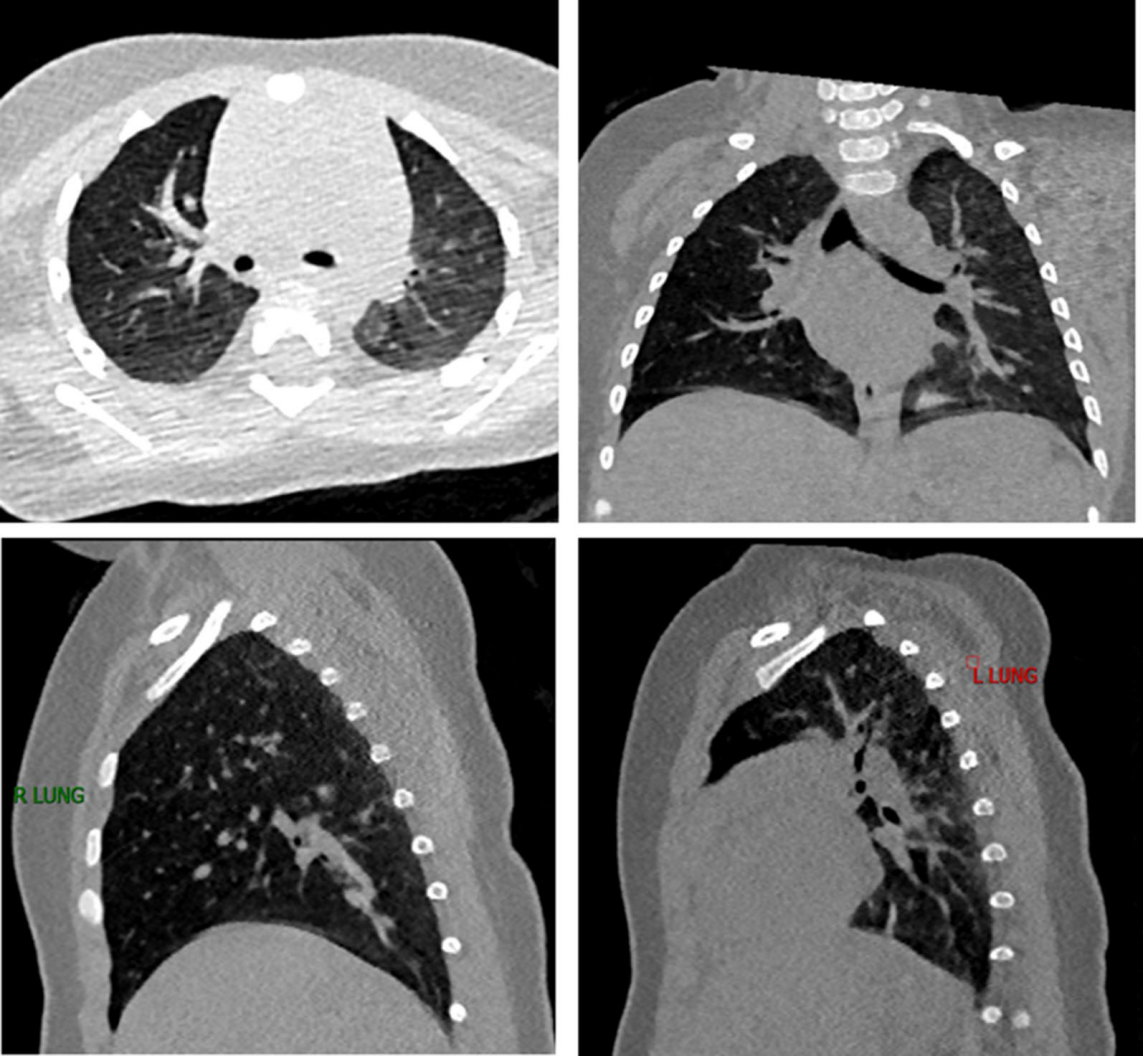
Chest CT image of patient A on April 12th. The posterior segment of the left upper lobe had limited “mosaic” signs, and the right side of the lung parenchyma was high
